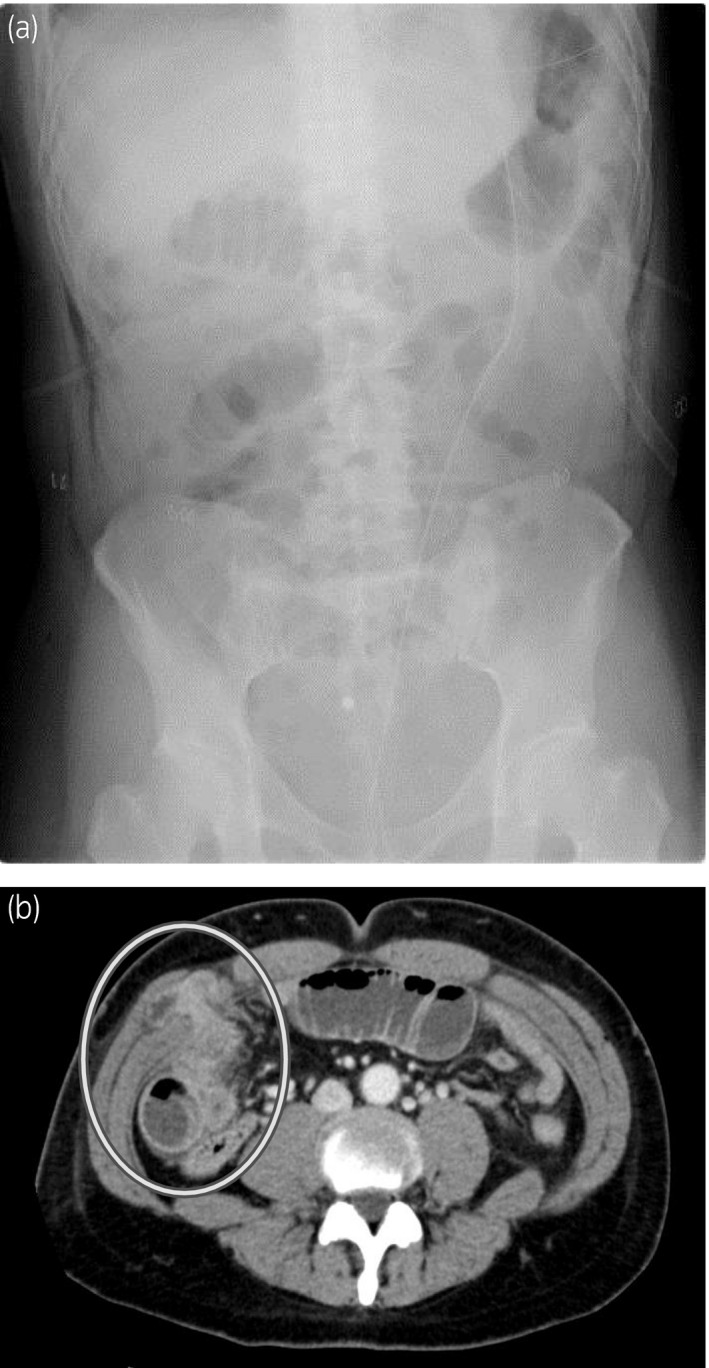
(a) KUB at the onset of hernia. The niveau sign was on the right side of the abdomen, suggesting intestinal obstruction, so we proceeded with a contrast‐enhanced CT scan to detect the cause. (b) Abdominal enhanced CT scan at the onset of hernia. The intestine had escaped from the peritoneum from the port site of the robotic right‐hand arm and the intestine on the oral side was expanding, which was diagnosed as a postoperative ileus due to port site hernia.
